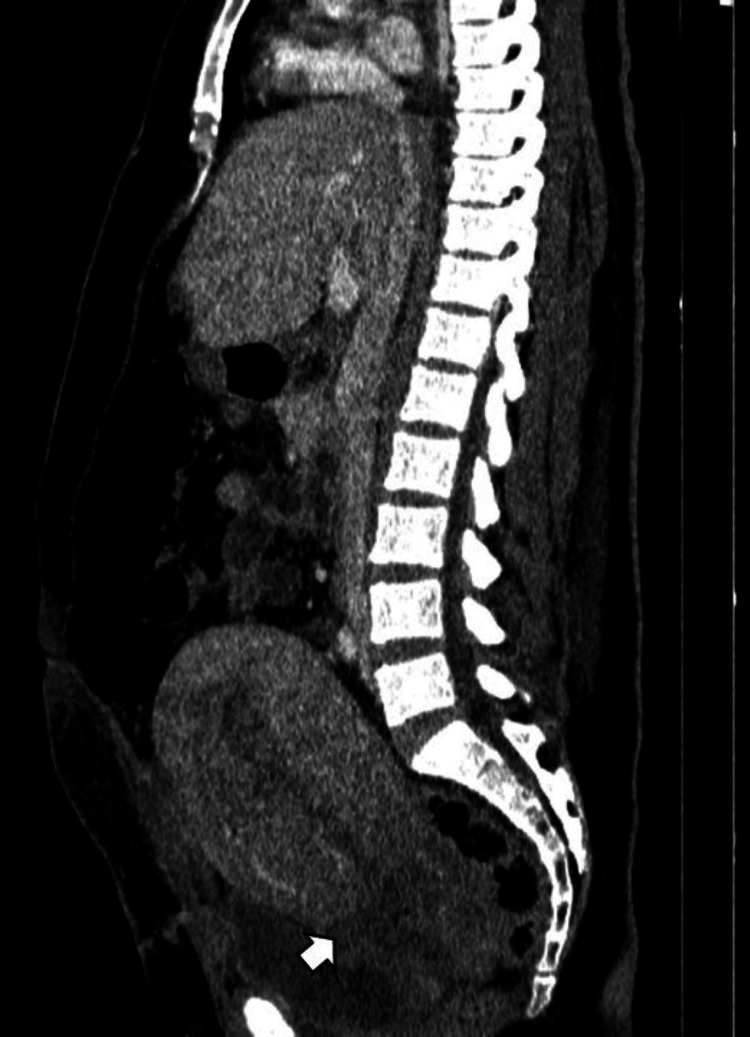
Sagittal contrast-enhanced CT image showing anterior abdominal wall edema and small postoperative air bubbles adjacent to the cesarean scar (white arrow). CT: computed tomography
